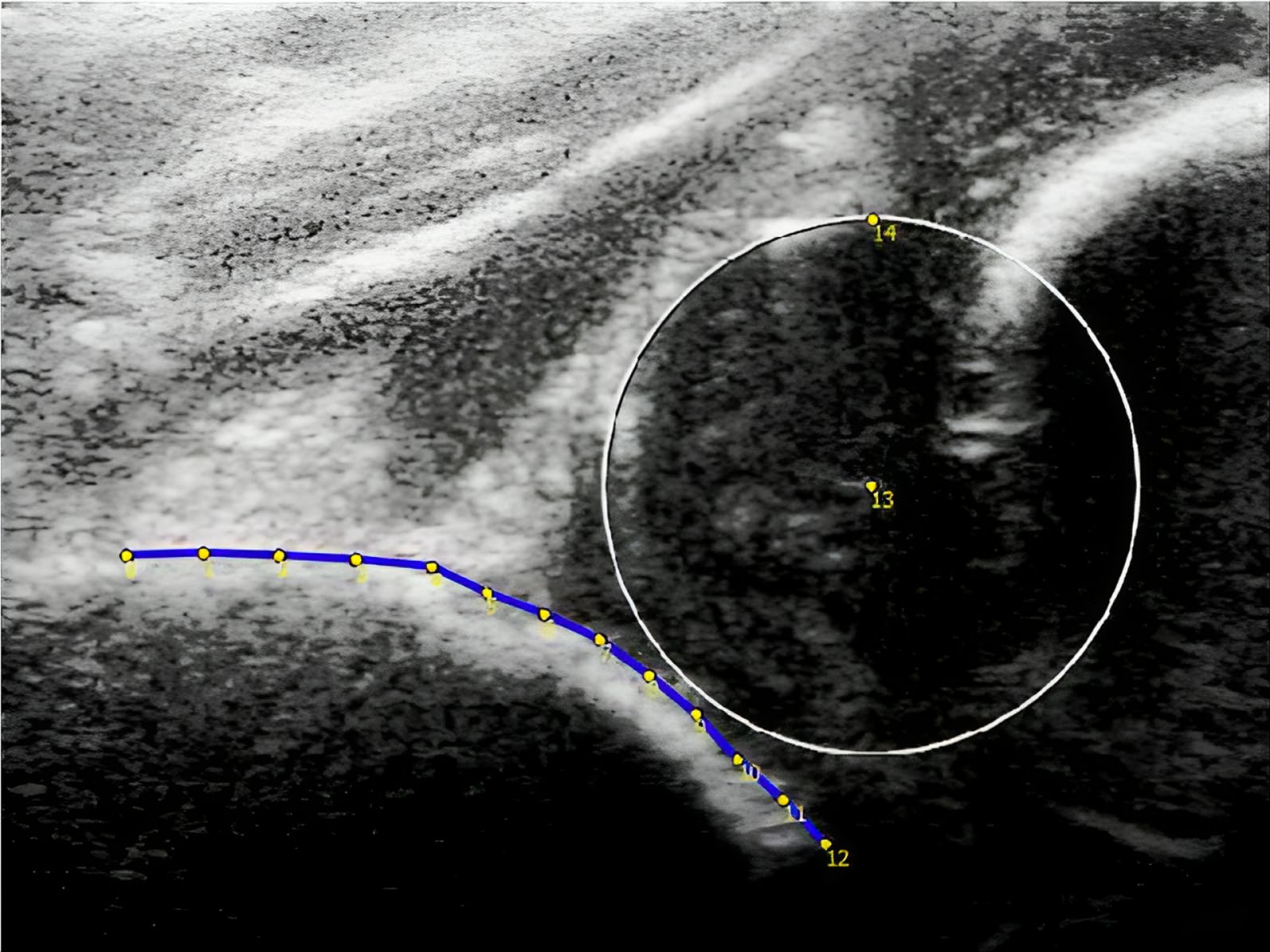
Baseline ultrasound of a decentered hip with points 0–12 at the bony contour of the ilium and acetabulum. Points 13 and 14 are placed on a best-fitted circle drawn over the femoral head
